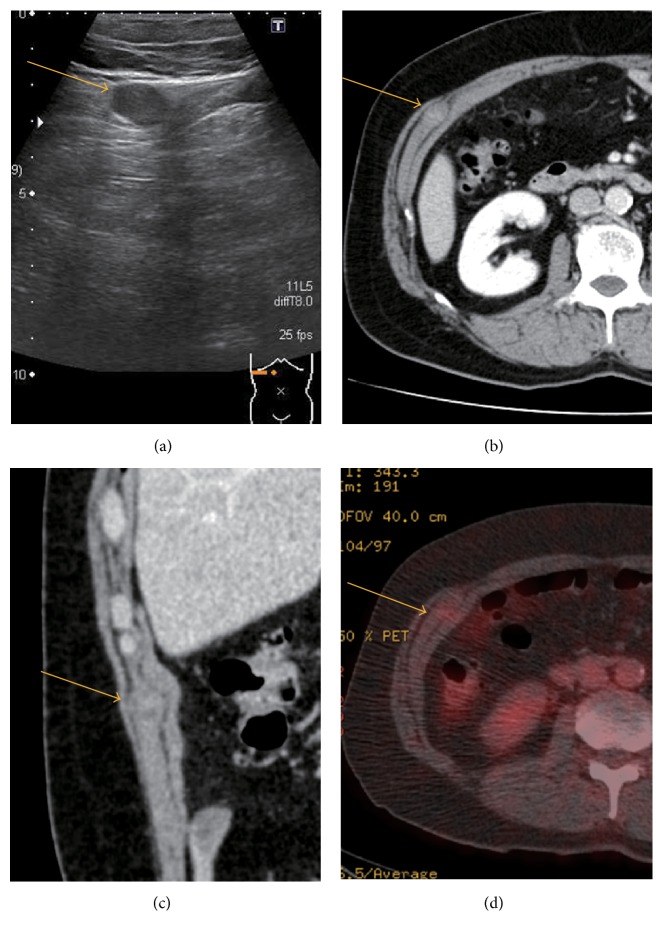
(a) US shows a well-demarcated hypoechoic mass in the abdomen. (b) Axial view and (c) coronal view of the CT abdomen with intravenous contrast. The mass shows a heterogeneous density in the internal abdominal oblique muscle. (d) FDG-PET demonstrated low FDG uptake in the tumor. The maximum SUV of the tumor was 1.4.
